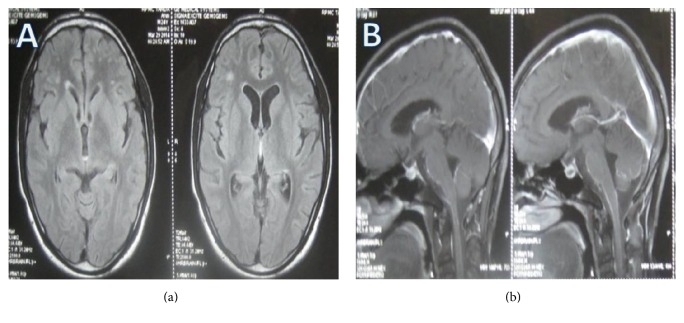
(a) Axial FLAIR images showing white matter periventricular and subcortical hyperintensities bilaterally. (b) After contrast brainstem meningeal enhancement is seen.
